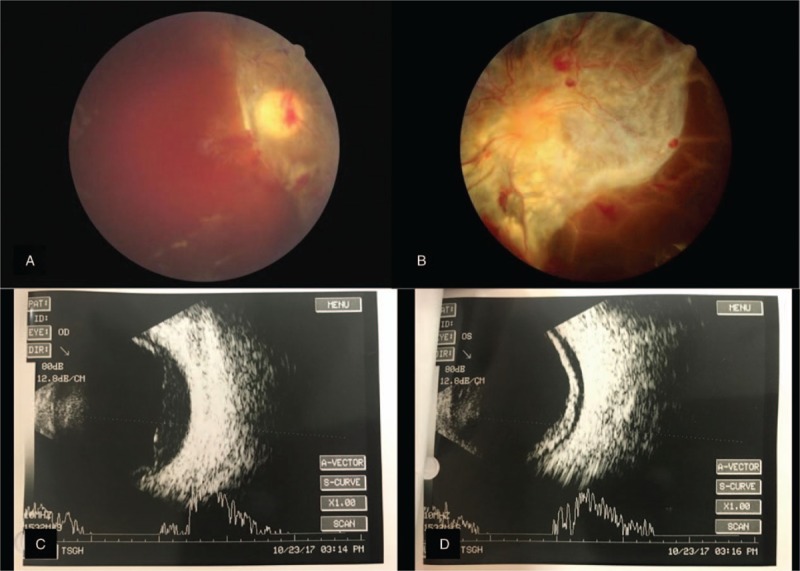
(A, B) Fundus photographs and (C, D) B-scan sonographic images of both eyes at 1 month after treatment with an intravitreal antivascular endothelial growth factor agent. Severe fibrovascular proliferation with hemorrhage and tractional retinal detachment are present in the right eye (A, C) and left eye (B, D).
